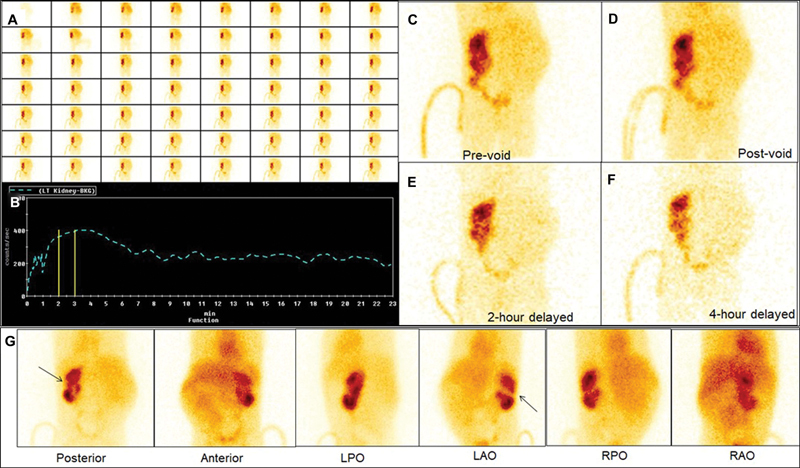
A 5-year-old boy with Prune belly syndrome presented with bilateral undescended testes, thinned anterior abdominal wall muscles, grossly dilated urinary bladder, and hypoplastic right kidney with impaired renal function test (serum creatinine 2.4 mg/dL), deranged hemogram (low hemoglobin), and elevated WBC count possibly due to urinary tract infection. Tc-99m EC dynamic renal scintigraphy (
